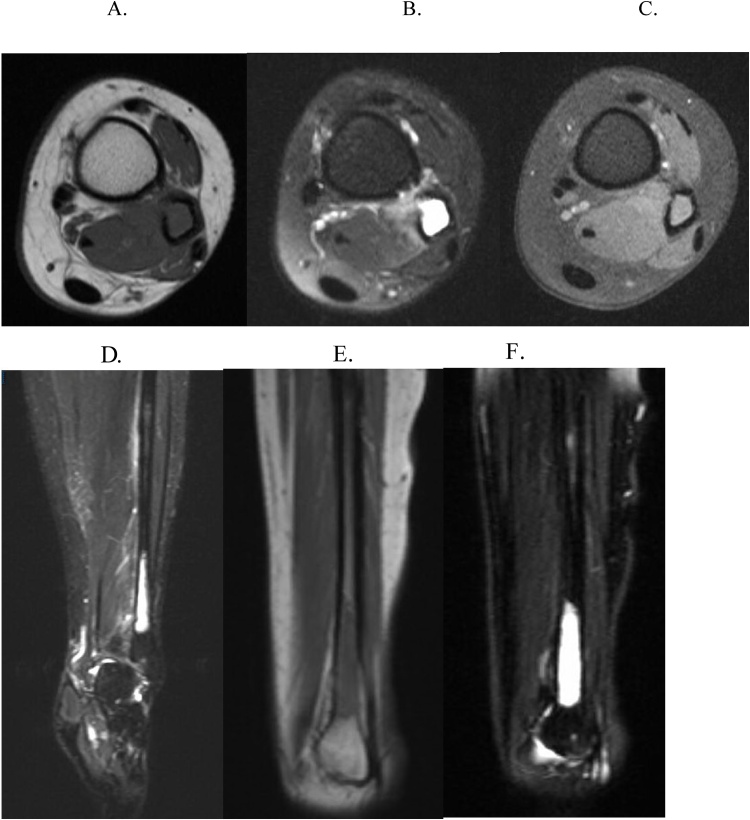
MRI of the left distal tibia/fibula in patient #2 after neoadjuvant chemo and radiation therapy. A) Axial T1. B) Axial T2 FS. C) Axial T1 FS. D) Coronal STIR. E) Sagittal T1. F) Sagittal T2 FS.
